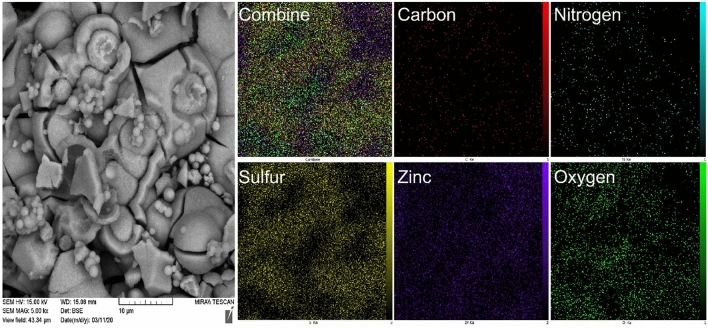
X-ray mapping analysis of Zn-CA-CPS.
